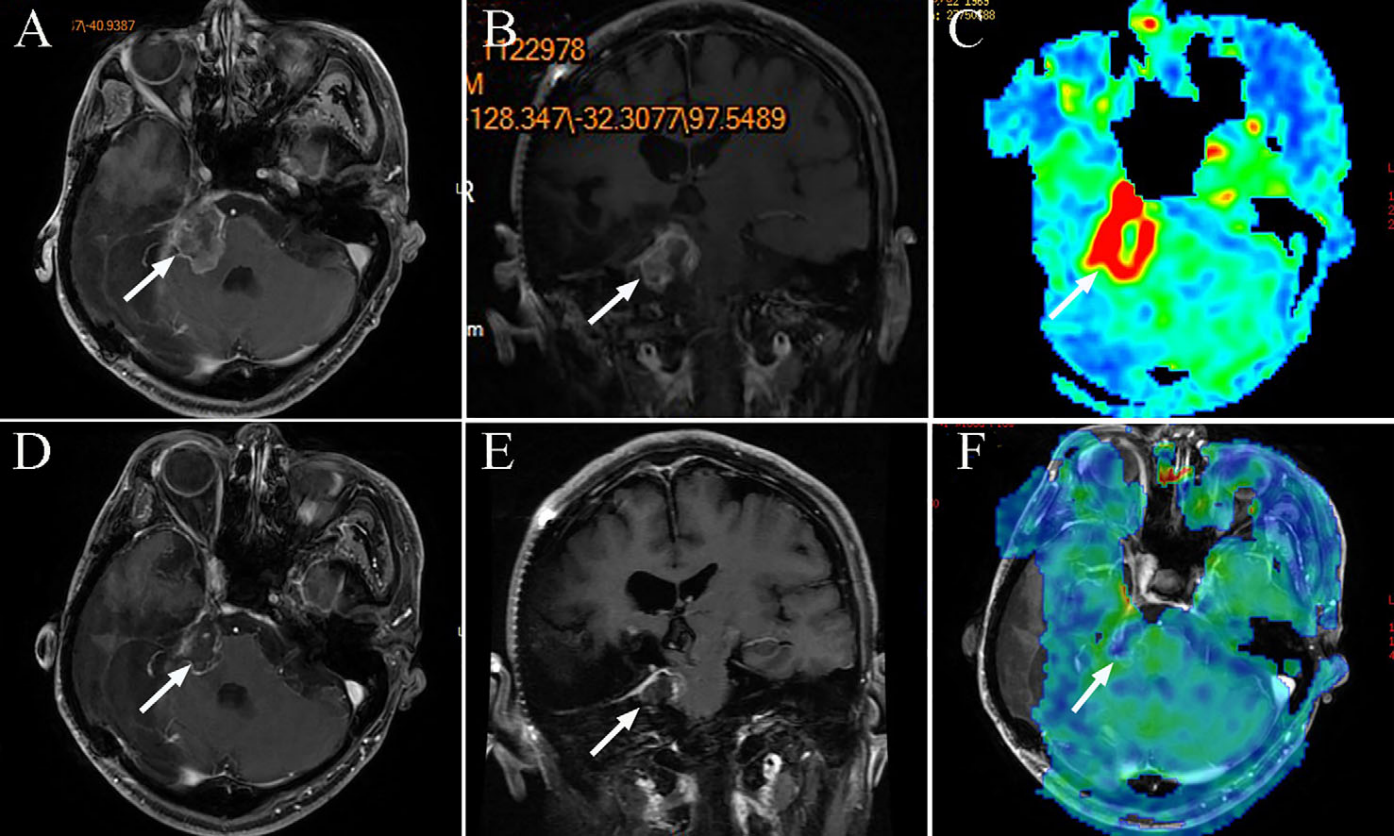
The MRI images of patient 9, tumor 20. (A) Axial T1-weighted contrast-enhanced image showing residual enhancement and a pretreatment tumor volume of 13.41 cm^3^ (tumor 20); (B) Coronal T1-weighted contrast-enhanced image; (C) Axial three dimensional arterial spin labeling (3D-ASL) image showing high perfusion; (D) Axial T1-weighted contrast-enhanced image after 12 months, the tumor reduced. (E) Coronal T1-weighted contrast-enhanced image after 12 months; (F) Axial 3DASL image showing low perfusion after 12 months.
